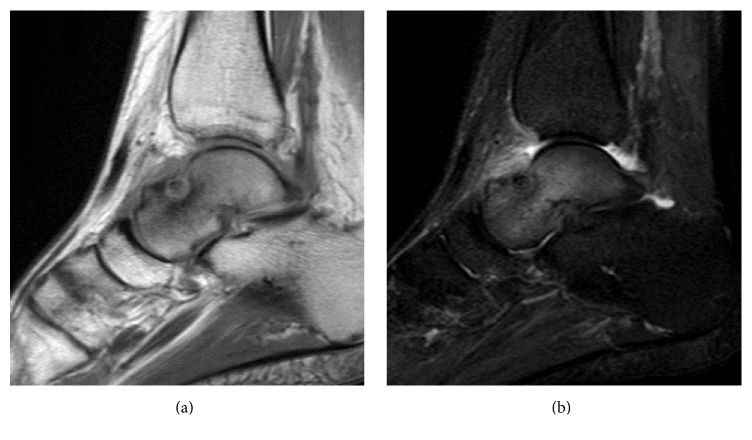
T1-weighted (a) and short T1 inversion recovery (b) sagittal magnetic resonance imaging showing a bone lesion in the talar neck with surrounding bone marrow edema and synovitis in front of the bone lesion.
